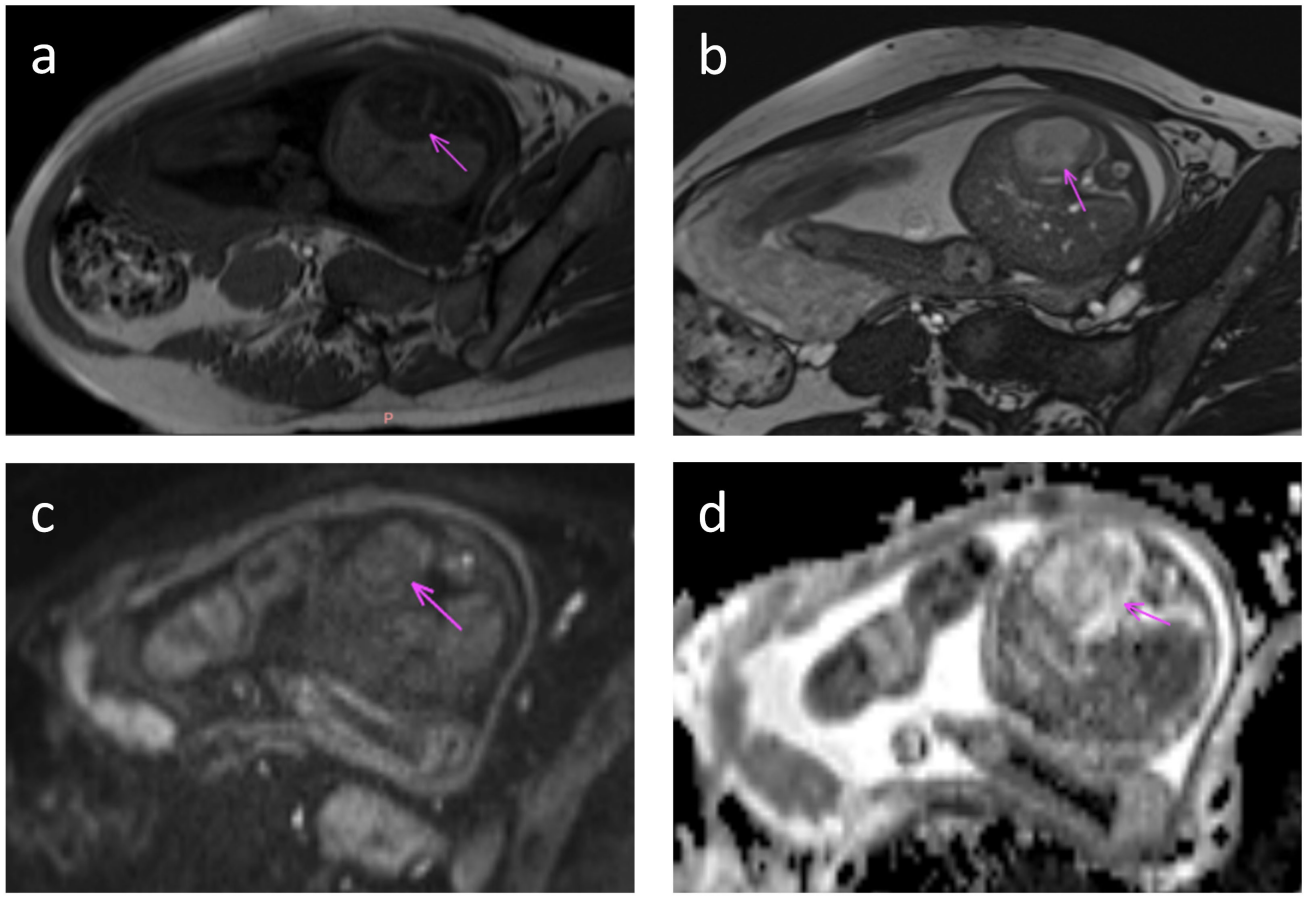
Fetal MRI at 36 weeks gestation. Axial MRI images through the fetal abdomen show a tumor in the left lobe of the liver (arrow). (a) T1-weighted image shows low T1 signal in the tumor. (b) T2-weighted image shows high T2 signal. (c) Diffusion weighted image with b value = 800 shows intermediate signal and (d) ADC map shows typical heterogeneous signal with areas of facilitated and restricted diffusion.
